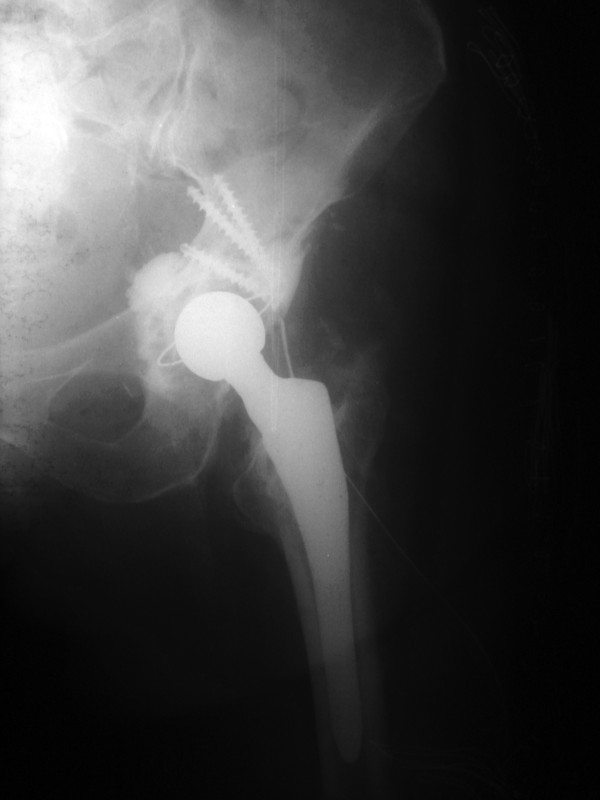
Postoperative x-ray after the total hip arthroplasty. A posterior-superior acetabular lip bone loss restored with hip autograft and antibiotic-impregnated cement fixed with three screws.
